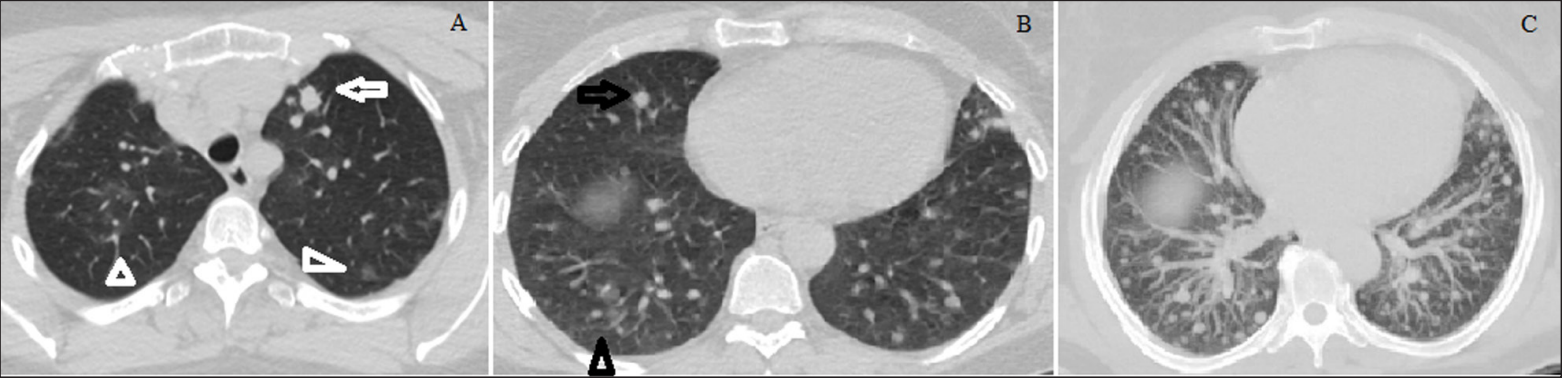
Baseline chest CT shows ground-glass opacities (GGO) (arrowheads) in the bilateral lung parenchyma and multiple solid nodules that the largest one has a diameter of 13 mm (arrow), which is at the anterior segment of the left upper lobe. (A). In the lower lobes, many solid nodules with a maximum size of 8 mm on the right upper lobe (black arrow) and GGO in the nodular form (black arrowhead) are observed (B). The Maximum Intensity Projection (MIP) image shows bilateral randomly distributed multiple solid nodules.
